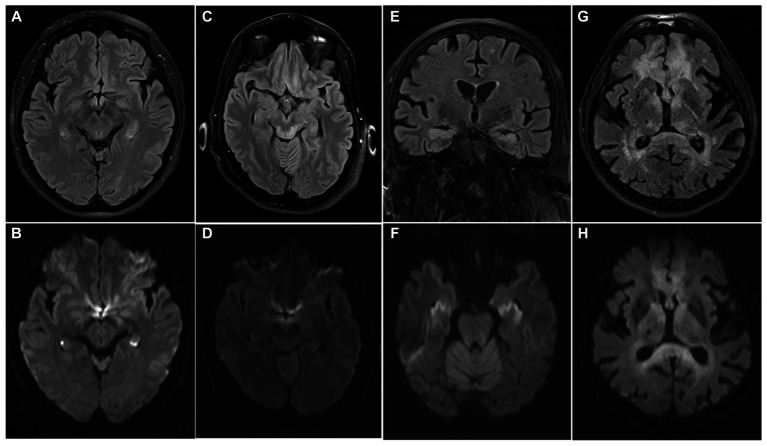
MRI differential diagnosis of acute amnestic syndrome. Transient global amnesia (A,B), Wernicke-Korsakoff syndrome (C,D), limbic encephalitis (E,F), and lymphoma (G,H) can present with acute anterograde amnesia, with or without other neurological symptoms. Bilateral HPDLs in a case of TGA are showed (A) (FLAIR) and (B) (DWI). Typical tectal plate lesions associated with alcoholic Wernicke-Korsakoff syndrome are showed in FLAIR and DWI sequences (C,D). MRI shows bilateral hyperintensity of the hippocampi on both FLAIR (E) and DWI (F) sequences in a case of paraneoplastic limbic encephalitis. A central nervous system lymphoma involving the fornix, but also frontal lobes and periventricular withe matter is showed in (G) (FLAIR) and (H) (DWI), appearing as a diffuse hyperintense alteration on both sequences.
